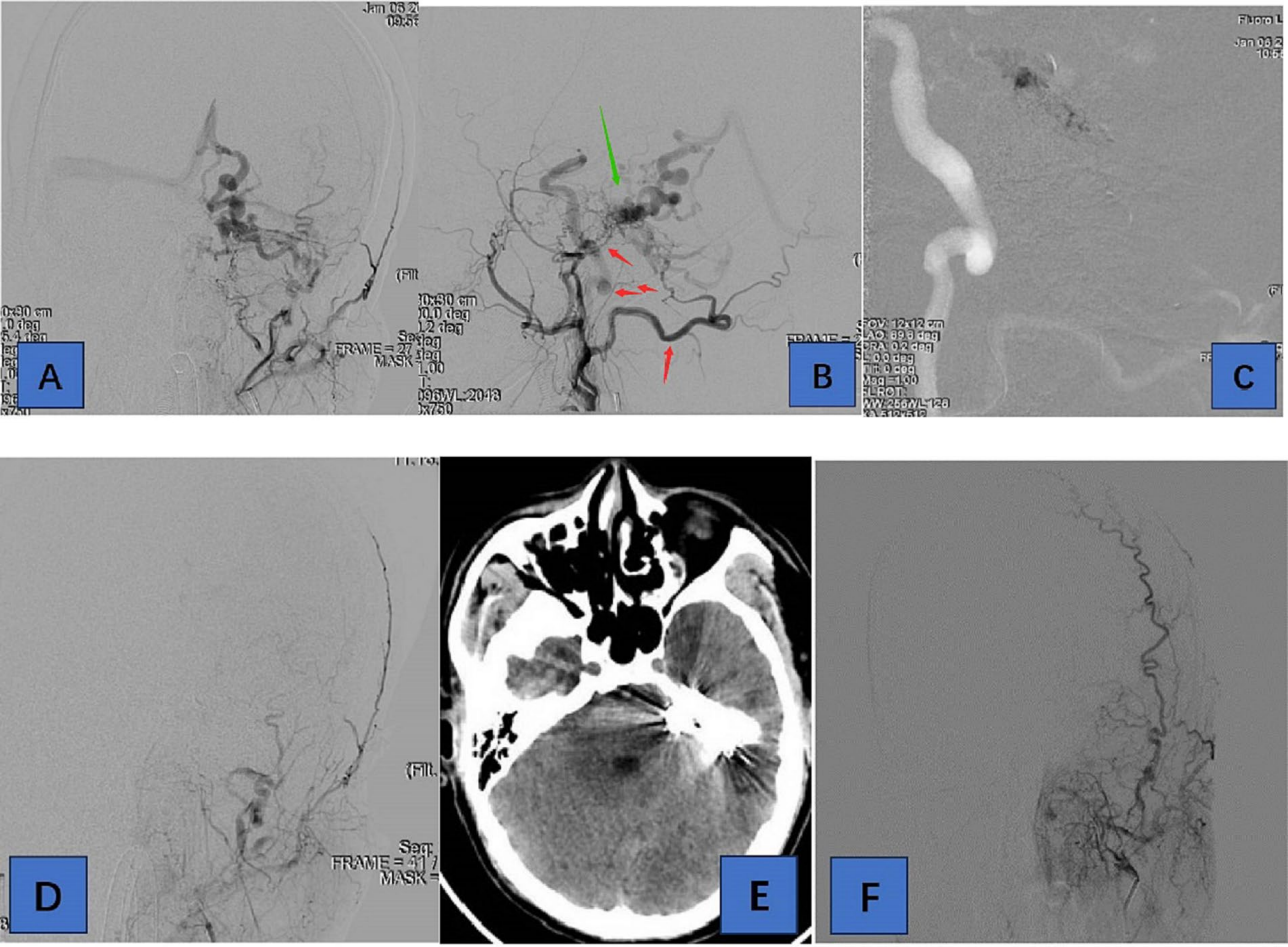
Adult patient presented with headache for 1 week, whose DSA suggested a tentorial dural arteriovenous fistula of type G6. (A,B) are the frontal and lateral views of the left external carotid artery, showing the left occipital, posterior auricular, middle meningeal, and ascending pharyngeal arteries involved in the blood supply. (C) We chose the left occipital artery route for embolization of TDAVF. (D) is a postoperative left external carotid arteriogram showing complete embolization of the TDAVF. (E) is a postoperative cranial CT showing hyperdense embolic shadow. In (F), there was no recurrence of TDAVF on 3-month postoperative angiography. Red arrows point to arteries, and green arrows point to TDAVF.
